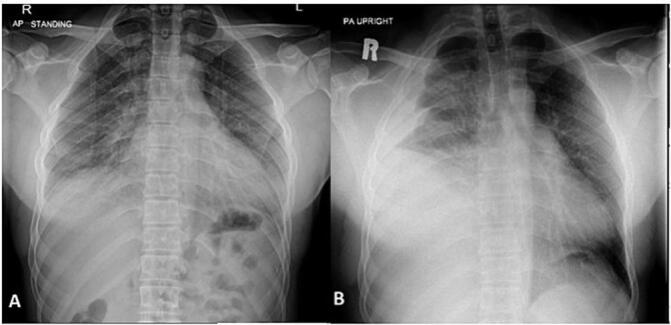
A - chest X-ray on initial admission. B – Chest X-ray on subsequent admission showing right sided effusion.
